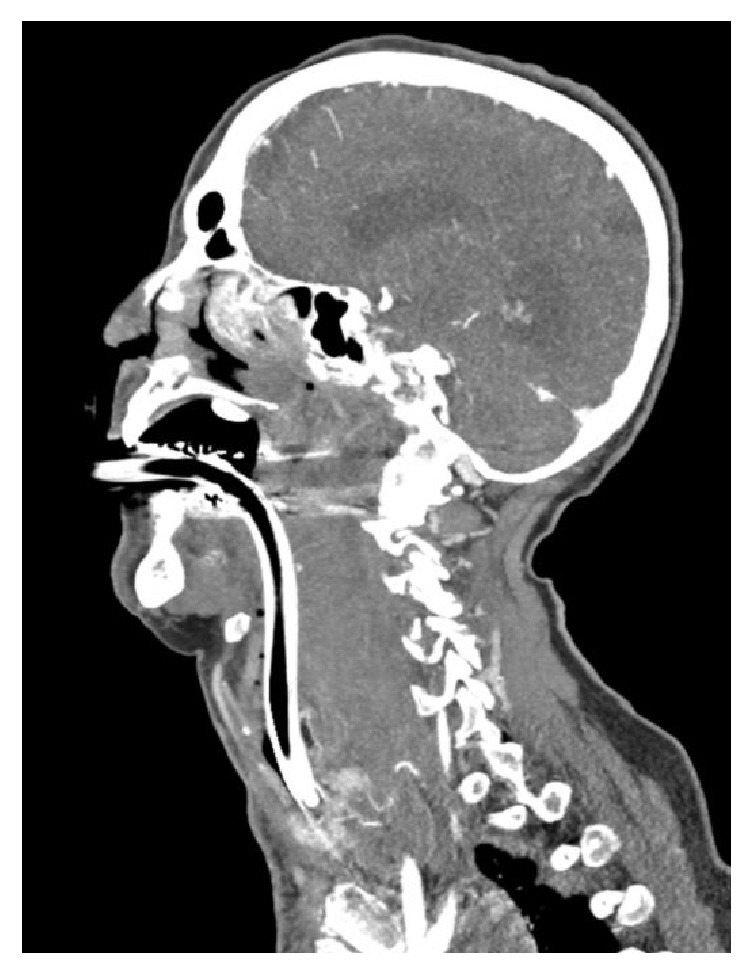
Sagittal plane CT angiogram of neck following intubation demonstrating a large retropharyngeal hematoma and anterior airway displacement.
